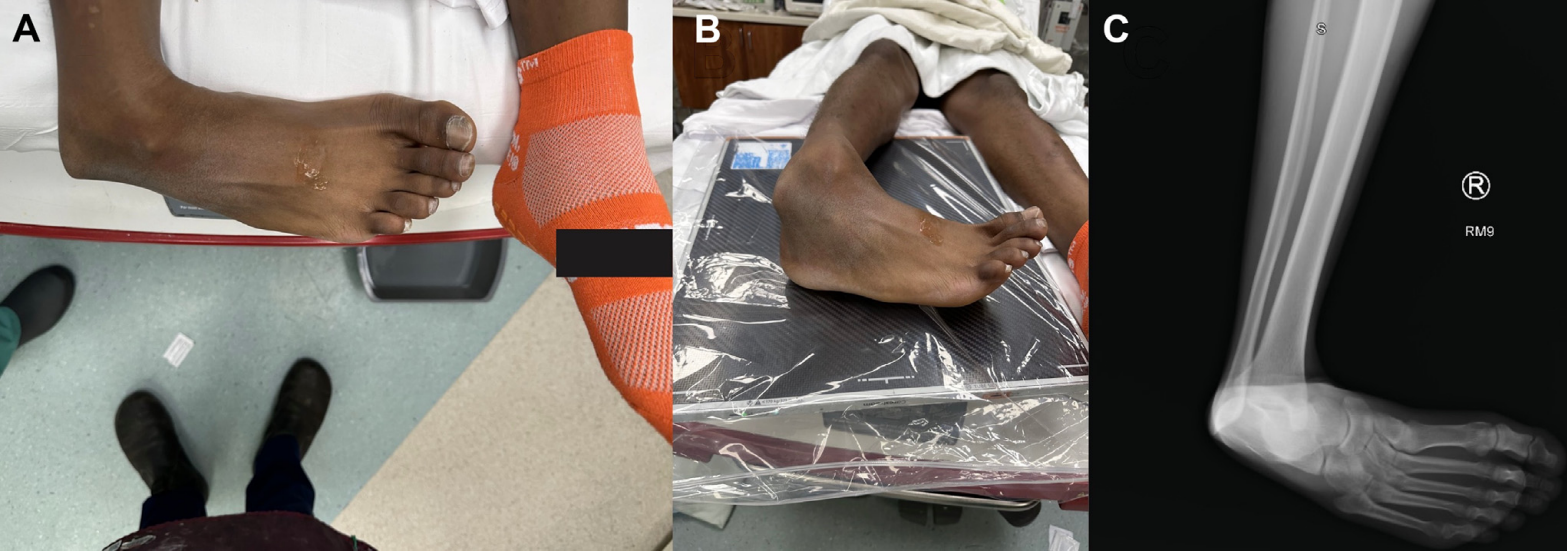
Visible deformity of the ankle with the foot positioned posterior to the ankle mortise with tenting of the skin (A, B). Anteroposterior radiograph of the right ankle showing a posterior ankle dislocation without fracture (C).
